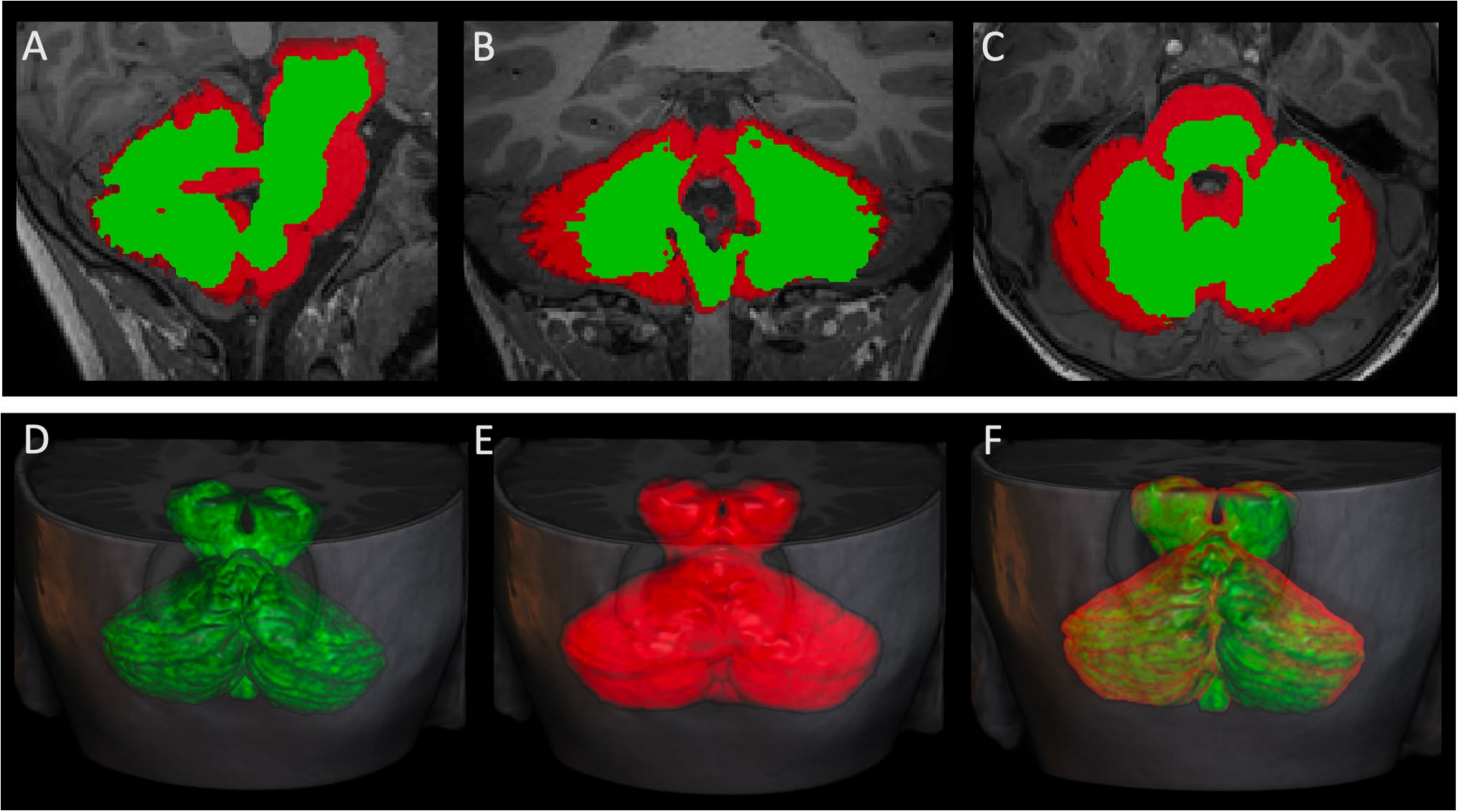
Comparison of the cerebellar volumes of the patient with an age-matched healthy subject. (A–C) Segmentation of the cerebellar volumes of the patient (green maps) and of an aged-matched control subject (red maps) overlayed on sagittal (A), coronal (B), and axial-reformatted (C) 3DT1-weighted images. (D–F) Volumetric reconstructions of cerebellar segmentations of the patient (green cerebellum, D), of the control subject (red cerebellum, E), and of the fusion of both cerebellar volumes (F) overlayed on 3D T1-weighted images. Note that the volume of both the cerebellar hemispheres and vermis of the patient is smaller compared to the age-matched control
